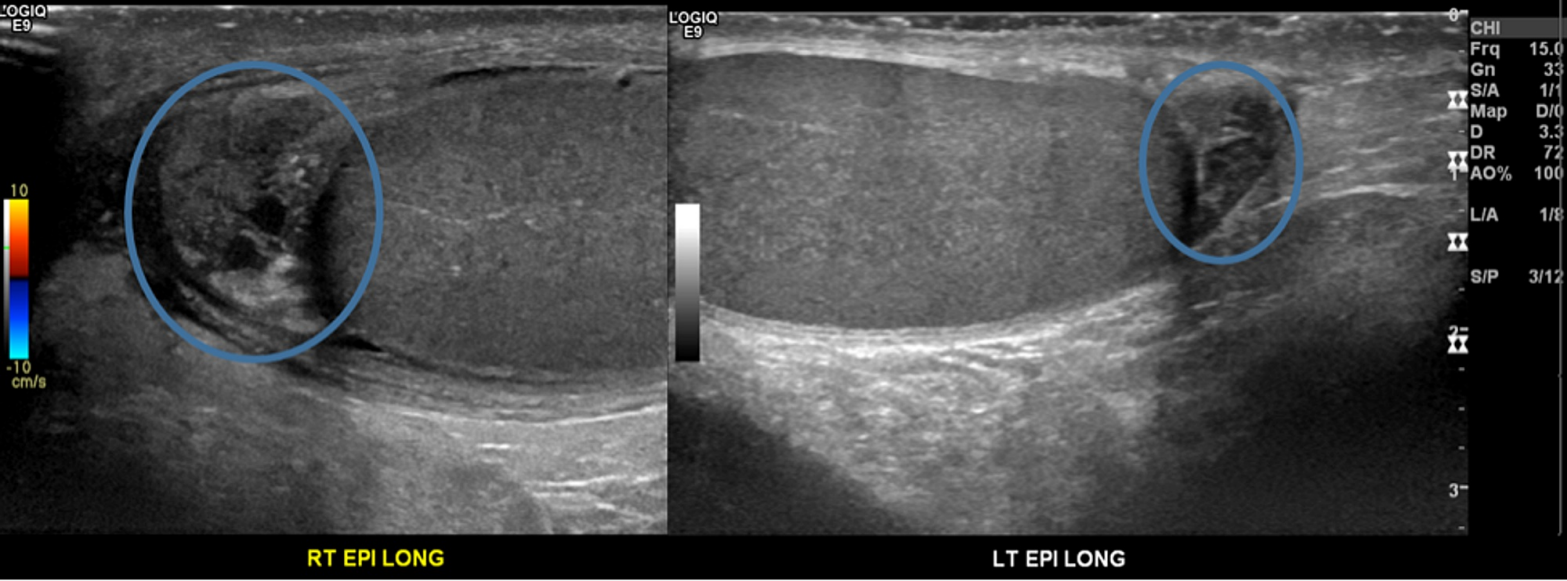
Long grey-scale ultrasonography of the bilateral epididymis Blue circles: slightly edematous right epididymis measuring 15 x 10 x 10 mm compared to the left epididymis measuring at 9 x 7 x 9 mm.
